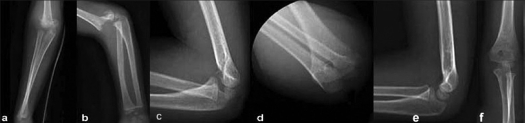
Pre-reduction anteroposterior (a) and lateral (b) radiographs of the elbow of a Gartland type-III fracture with pure posterior displacement. Post-reduction radiographs lateral view (c) and anteroposterior (d) view immobilized by collar cuff and cast around the wrist. Lateral (e) and anteroposterior (f) radiograph of the elbow at eight months follow-up
